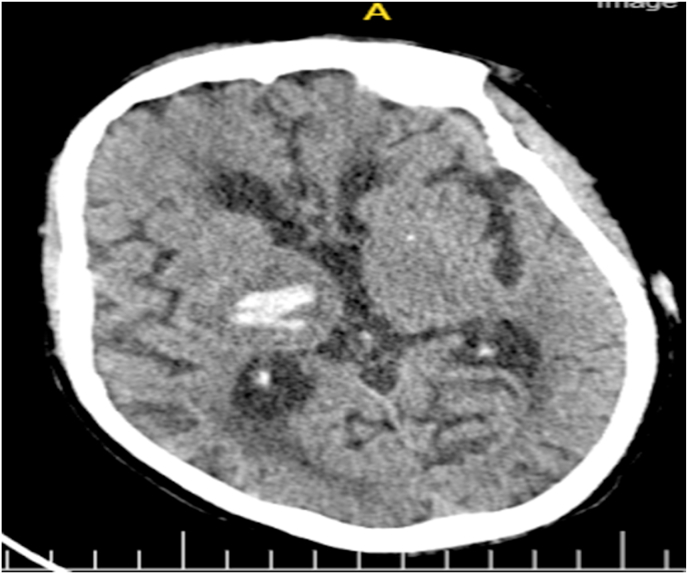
Head CT shows 3 cm diameter hyperdense parenchymal hematoma in the rightperiventriculary and basalganglia. And bifrontal periventricular diffuse hypodensity suggestive of chronic ischemiacchanges
